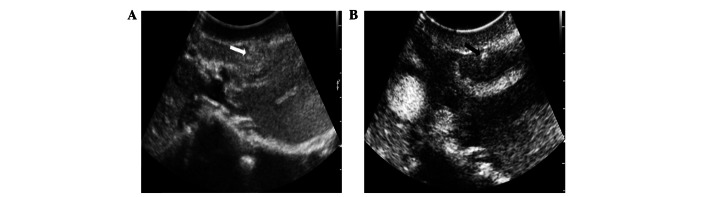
Images of a superficial laceration. (A) The parenchyma of the pancreas (white arrow) was inhomogeneous in the US image. (B) A superficial laceration of the pancreas (black arrow) was clearly shown by CEUS. The laceration was highly enhanced in the CEUS image due to active bleeding. US, ultrasound; CEUS, contrast-enhanced ultrasonography.
